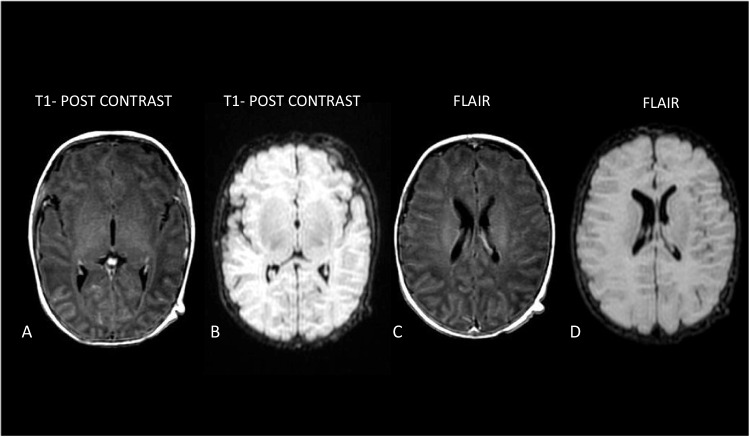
A, B Axial post-contrast T1-weighted images demonstrating diffuse leptomeningeal enhancement. C, D Corresponding axial FLAIR images obtained before contrast administration showing absence of sulcal hyperintensity or parenchymal abnormalities. Axial multiplanar reconstructions were derived from a sagittal 3D fat-suppressed FLAIR sequence obtained before contrast administration. Diffusion-weighted imaging showed no areas of restricted diffusion. ADC maps are not shown
